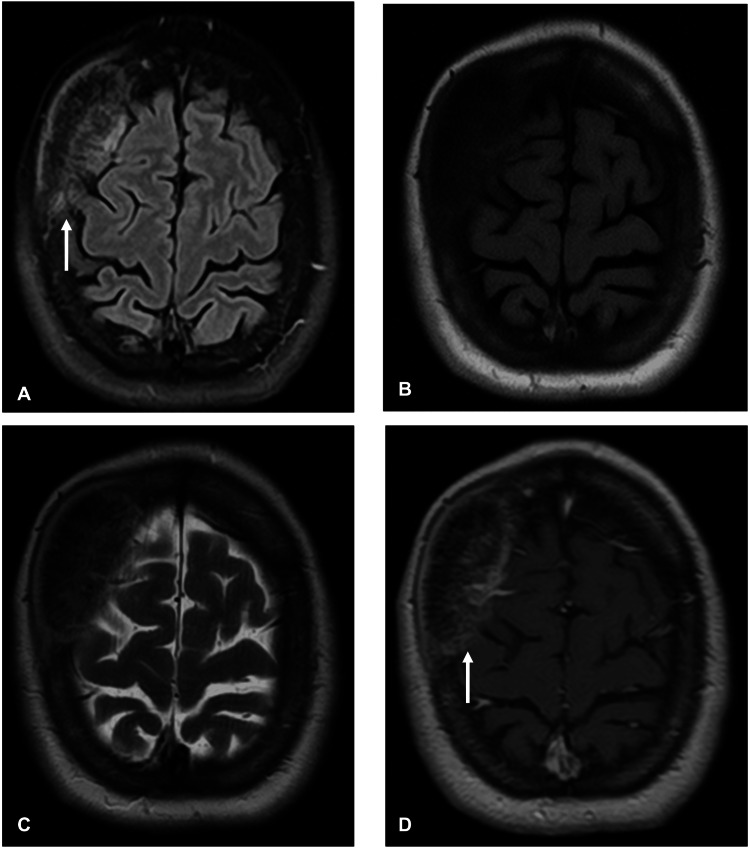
Contrast brain MRI. A. FLAIR image representing hyperintensity and thickened part of the affected skull in comparison to adjacent bone tissue. B. T1-weighted image showing no hyperintensity without contrast administration C. T2-weighted image showing isointensity D. T1-weighted contrast image showing contrast enhancement in a well-defined lesion, with well-delineated margins.
